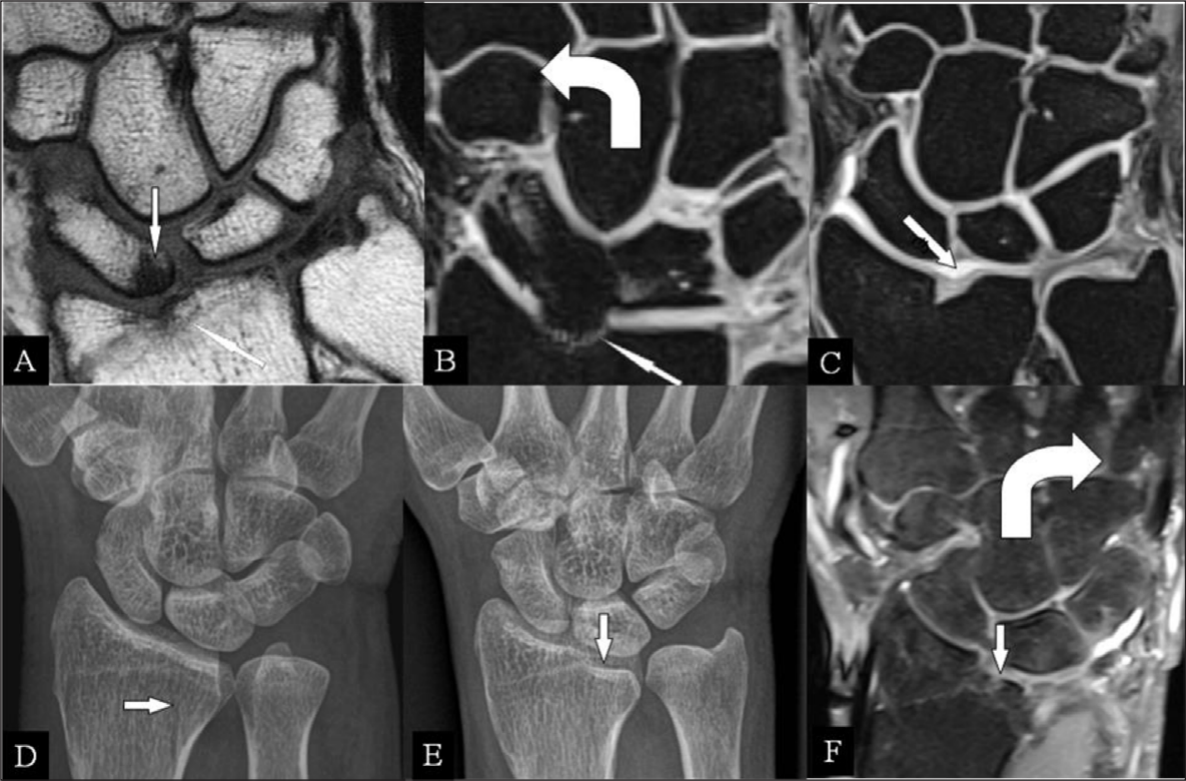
Radioscafoid and radiolunate abutment. (A) Coronal SE T1-WI; (B, C) Coronal 3D-GRE; (D, E) PA plain radiographs; and (F) Coronal SE PD-WI FS. (A) Sequela of an intra-articular fracture of the distal radial epiphysis with a residual step-off (oblique arrow) and marrow oedema (vertical arrow) at the proximal pole of the scaphoid bone. (B) Centrally, the cartilage is destroyed and the radial deviation is blocked. (C) Cartilage step-off in another patient. (D) The parasagittal intra-articular fracture was initially missed. (E) Consolidation with a depressed part of the articular surface. (F) Radiolunate abutment with blocked ulnar deviation.
